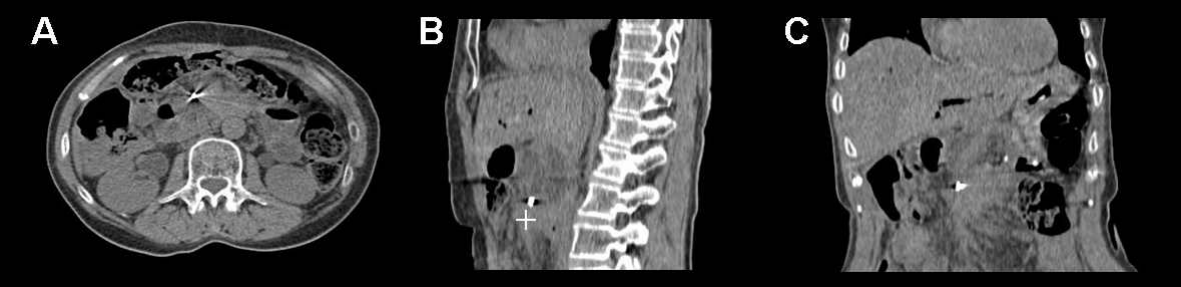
Axial (a), sagittal (b), and coronal (c) views of patient #10 at 0% expiration, acquired by 4D CT. The fiducials were always clearly distinguishable. The corresponding position of the fiducial at 100% inhalation is also shown (symbol ‘+’) in the sagittal section.
